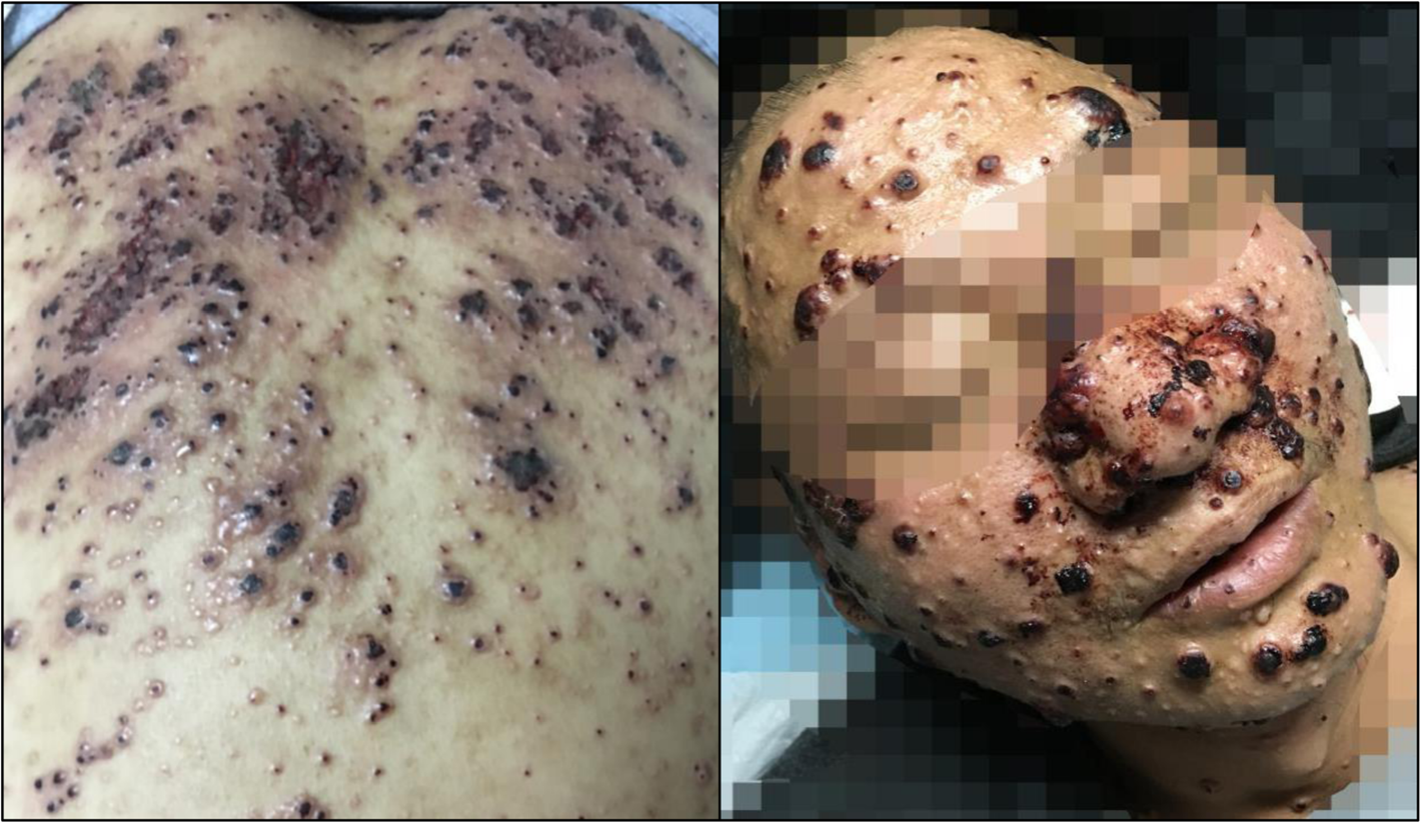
Chest CT of the patient showed features of pulmonary infection, with localized emphysema at the right lower lung, and multiple mediastinal lymphadenopathy
